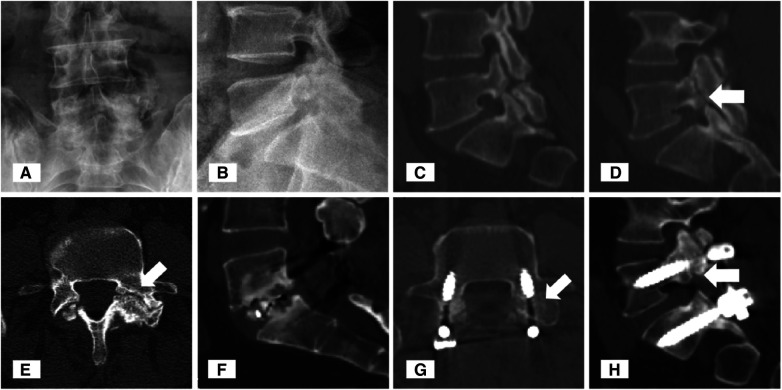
Typical images of Case 1. An anteroposterior plain radiograph revealed the obscure boundary of the left L5 pedicle (A). Lateral plain radiograph revealed Meyerding grade 1 spondylolisthesis (B). Sagittal and axial computed tomography (CT) images of the lumbar spine showed right spondylolysis (C) and right pediculolysis [(D,E), white arrowheads] of the L5 vertebra. Postoperative CT demonstrated that bony fusion occurred at the L5-S1 interbody space (F) and at the preoperative site of pedicle cleft (G,H).
